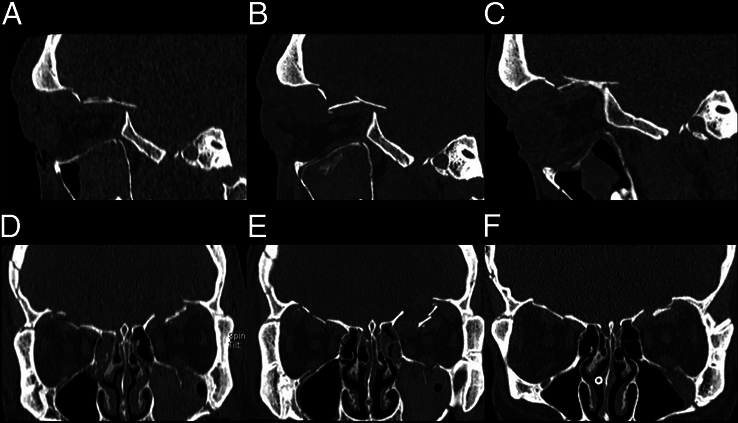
Computed tomography (CT) scans of the described case on day 0 (A, D), day 5 (B, E), and day 18 (C, F) demonstrating extensive facial fractures and evolution of left orbital roof position.
